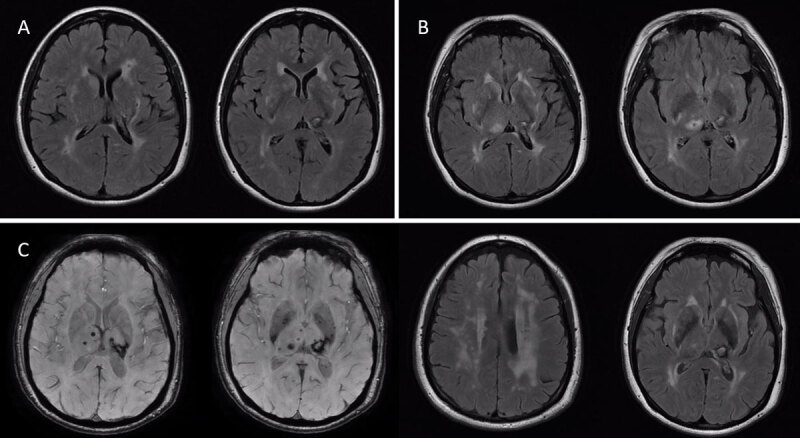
Axial brain MRIs performed at ages 46 (A), 47 (B), and 49 (C) years demonstrating the evolution of neuroimaging findings over time, including hemorrhagic subcortical lesions, especially in the left thalamus, and subcortical white matter lesions (A and B, axial FLAIR sequences and C, axial SWI and FLAIR sequences).
